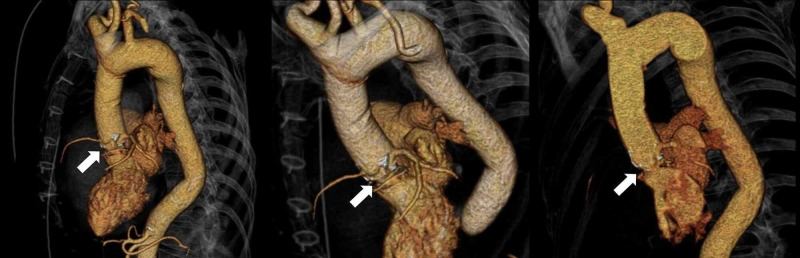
Pre-procedure three dimensional (3D) CT scan reconstruction, showing a luminal narrowing near the neo-Valsalva sinuses, which created measurement and planning difficulties
